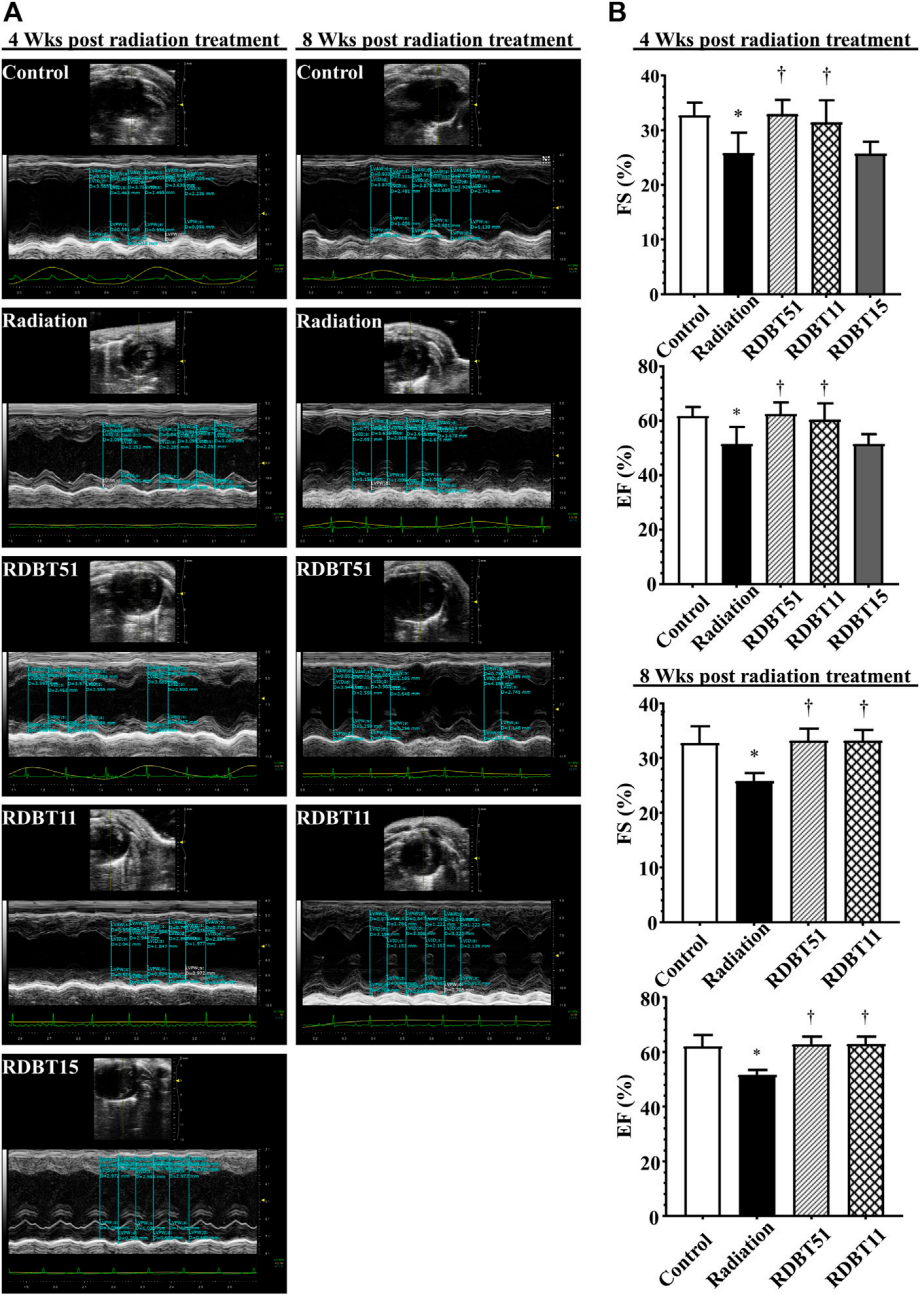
The DBT improves radiation-induced left ventricular systolic dysfunction. (A) Representative echocardiograms obtained at 4 and 8 weeks after radiation treatment; (B) Quantitative statistical analyses of FS and EF. The other concerning quantitative statistical analyses of cardiac function related parameters are summarized in Table 1. DBT, Dang Gui Bu Xue Tang; EF, ejection fraction; FS, fractional shortening; RAM, *Radix Astragali membranaceus*; RAS, *Radix Angelicae sinensis*; RDBT51, Radiation with DBT (RAM: RAS = 5:1); RDBT11, Radiation with DBT (RAM: RAS = 1:1); RDBT15, Radiation with DBT (RAM: RAS = 1:5).
